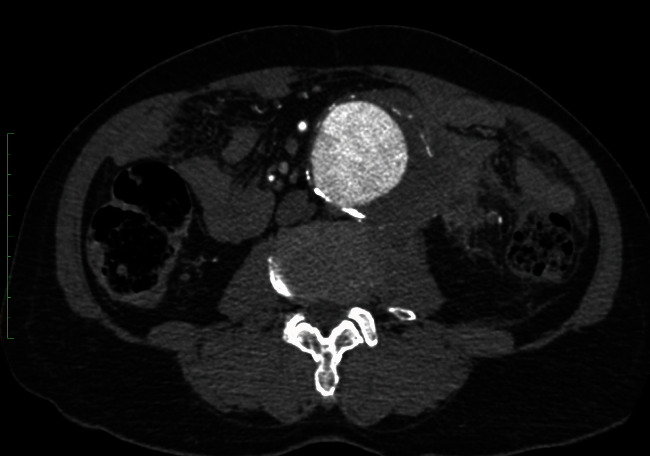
Preoperative CT scan revealed a large AAA with periaortic fat stranding.
